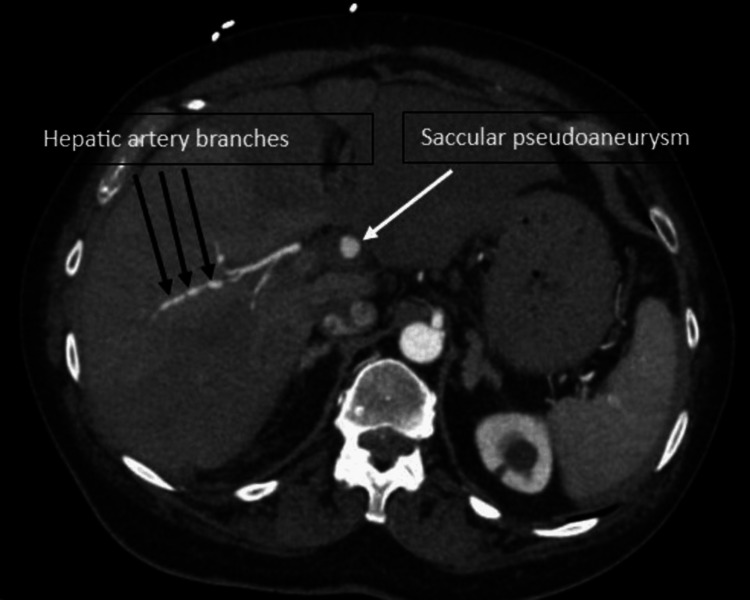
Proper hepatic artery pseudoaneurysm Axial contrast-enhanced CT shows saccular pseudoaneurysm of the proper hepatic artery (white arrow) and the bead-like irregularities of the hepatic artery branches (black arrows).
